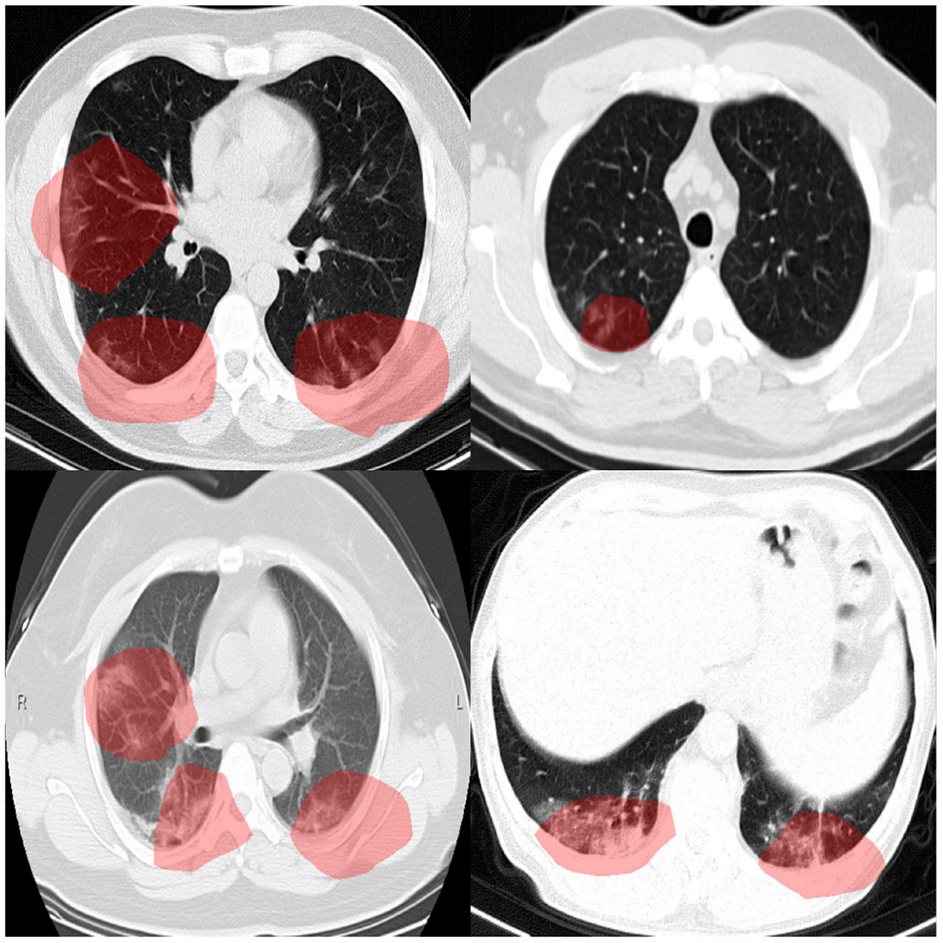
Example chest CT images from four COVID-19 cases, and the associated critical factors (highlighted in red) as identified by GSInquire (33) for COVID-Net CT-2 S.
